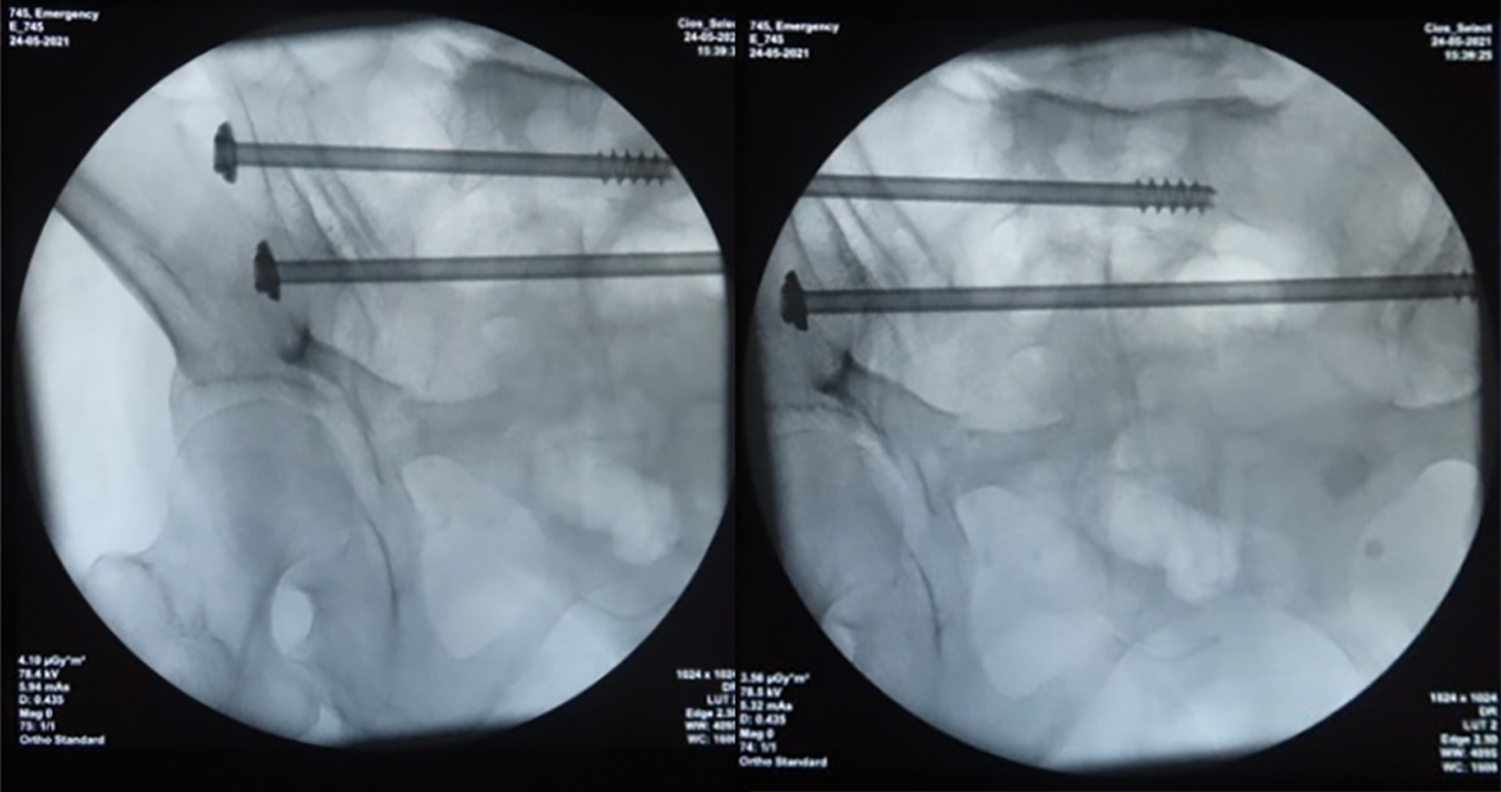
Radiographic imaging with C-arm.
